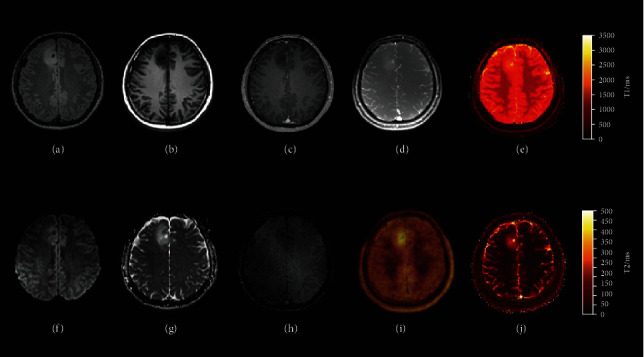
FET PET with MRI fingerprinting (MRF). Multiparametric ^18^F-FET PET-MRI and MR fingerprinting of a patient with a low-grade (WHO II) astrocytoma. (a) 3D FLAIR SPACE (sampling perfection with application optimized contrasts using different flip angle evolution) image. (b) T1-weighted MRI. (c) Postcontrast T1-weighted MRI. (d) MRF M0. (e) MRF T1-weighted image. (f) B1000. (g) ADC. (h) SWI. (i) PET/postcontrast T1-weighted MRI fusion. (j) MRF T2-weighted image. Reproduced with permission from Figure 8 of Haubold et al. [70].
